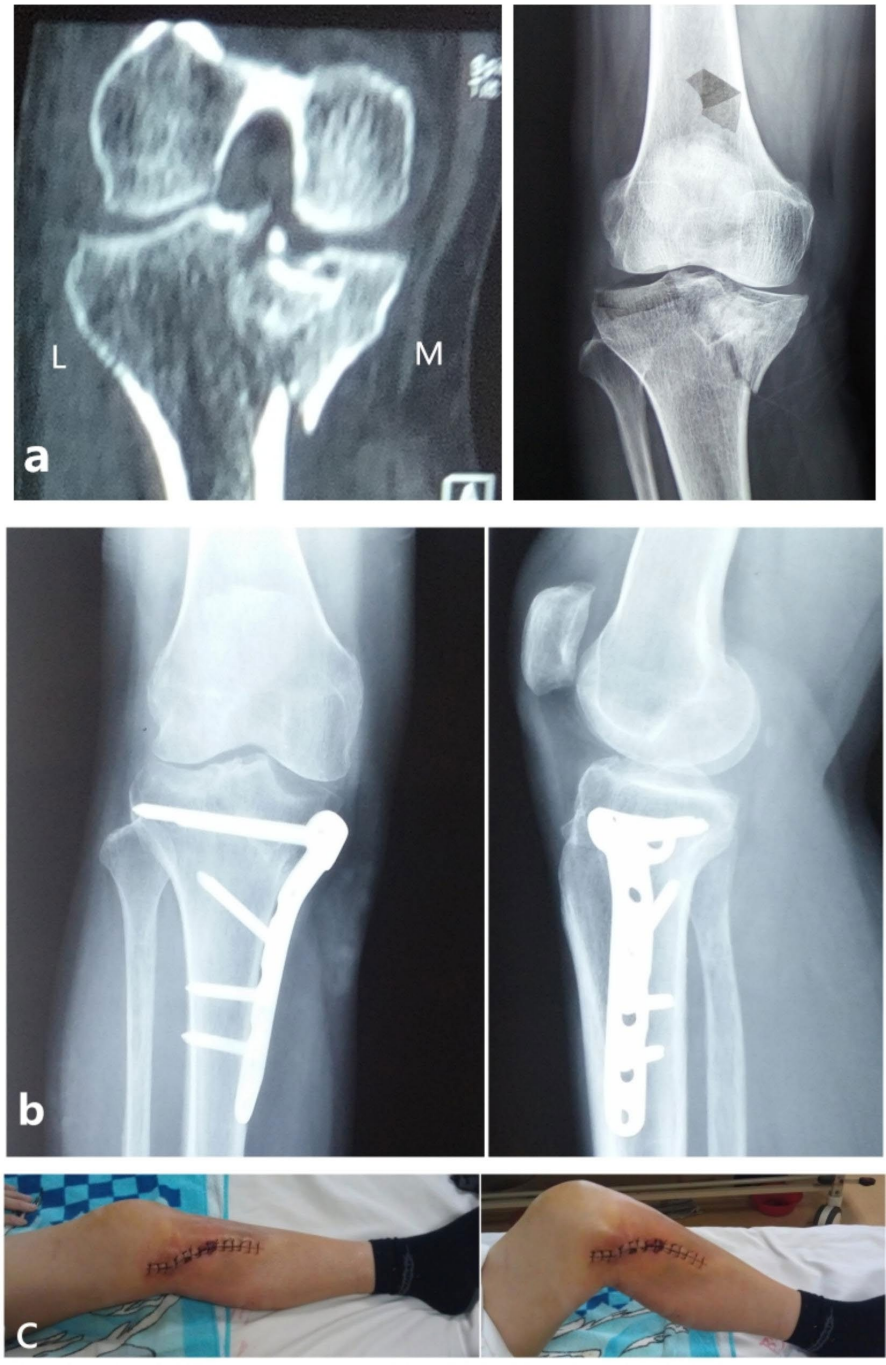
A typical case of a 45-year-old male with Schatzker IV tibial plateau fractures (extension-varus type). (a) The features of this type were shown in CT images. (b) After the reduction of MFA, a plate was needed for internal fixation. (c) Postoperatively, the function of the knee was good
